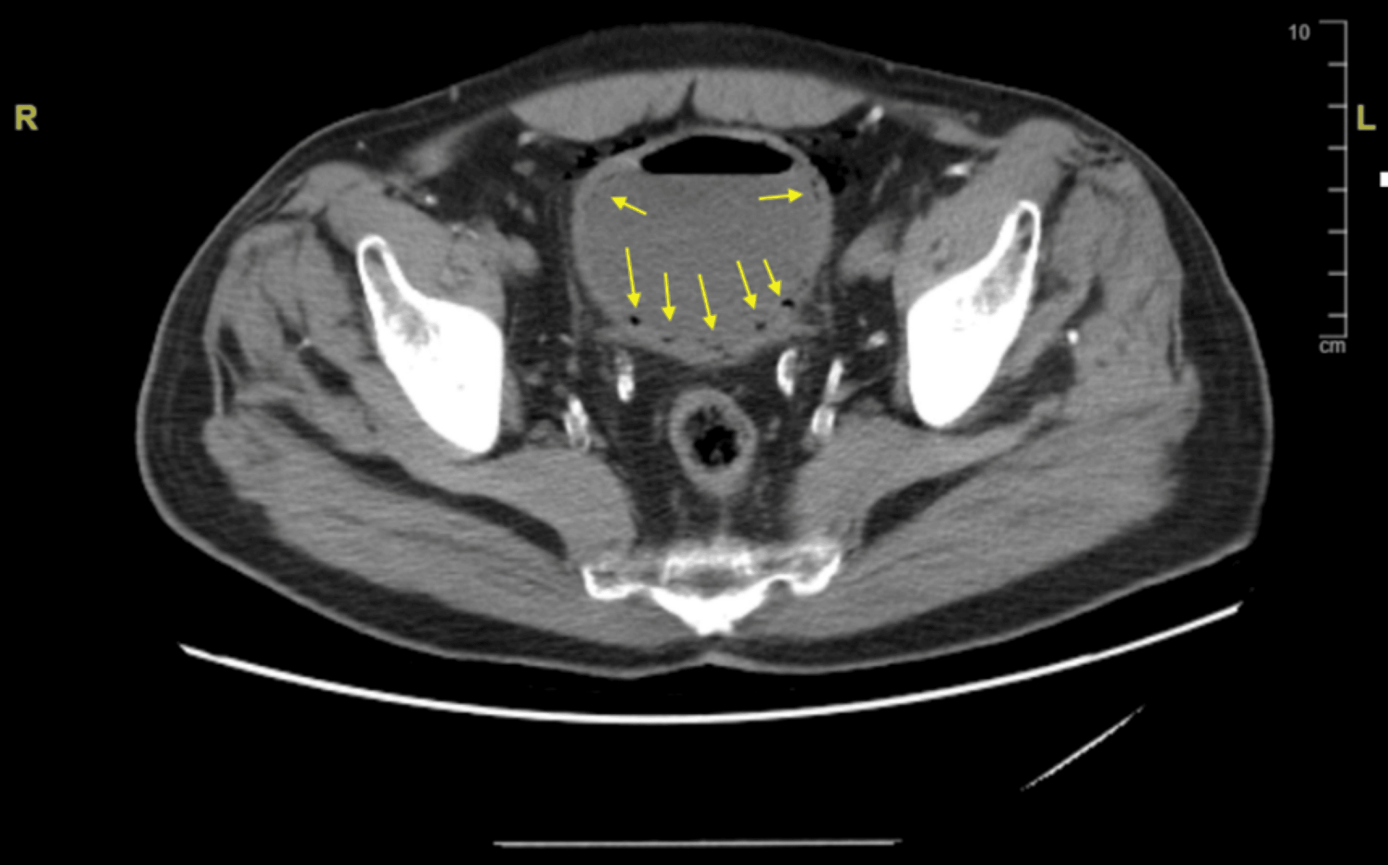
Computed tomography of the abdomen and pelvis The presence of air (yellow arrows) within the urinary bladder wall demonstrates findings consistent with emphysematous cystitis.
